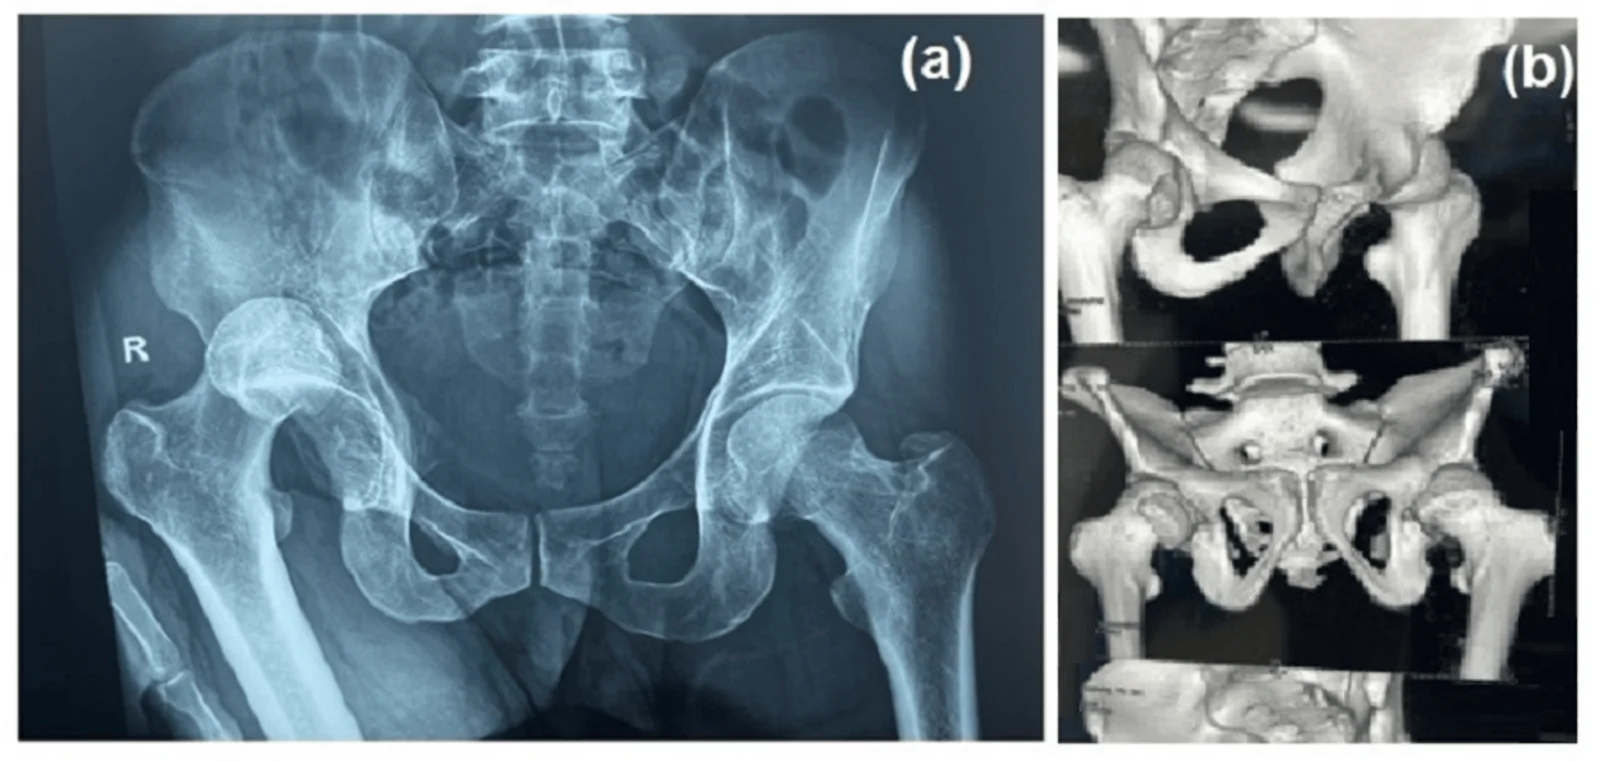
Case 2: Preoperative evaluation. (a) Anteroposterior pelvic radiograph at presentation demonstrating posterior dislocation of the right hip. (b) Three-dimensional CT reconstruction obtained after reduction demonstrating a Pipkin type II femoral head fracture involving the weight-bearing surface.
